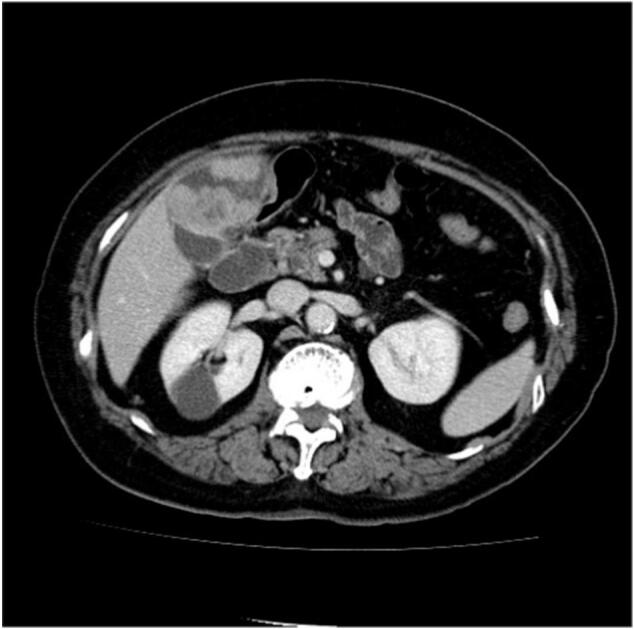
Computed tomography imaging taken 1 month before surgery.
